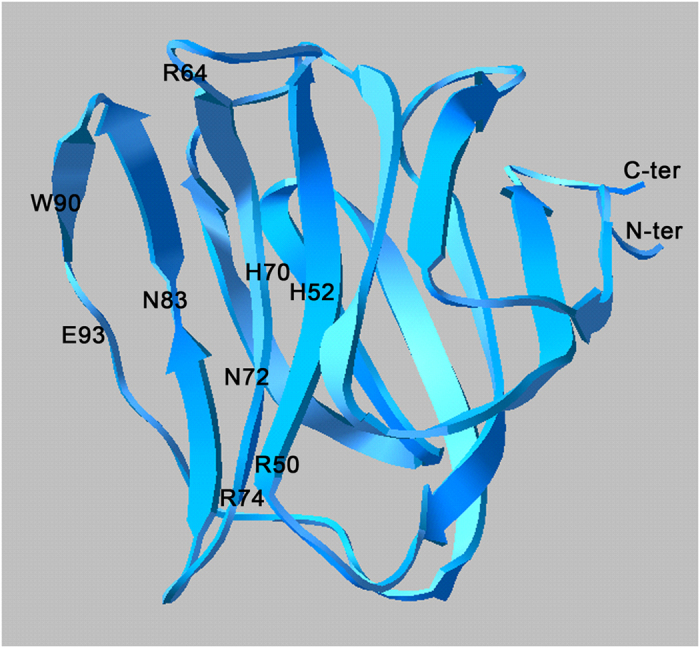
Three dimensional structure model of *Pl*-GAL-8 N-terminal domain. Ribbon model of *Pl*-GAL-8 N-terminal domain obtained by homology modelling using as template the X-Ray structure of the human Gal-8 N-terminal domain (pdb file: 2yv8;). The indicated amino acid residues are carbohydrates-binding sites known^23^ to interact with lactose via hydrogen bonding (R50, H70, N72, R74, N83, W90 and E93) or water-mediated bonds (H52 and R64) and with the galactose ring by *van der Waals* interactions (W90). N-ter, N-terminal; C-ter, C-terminal.
